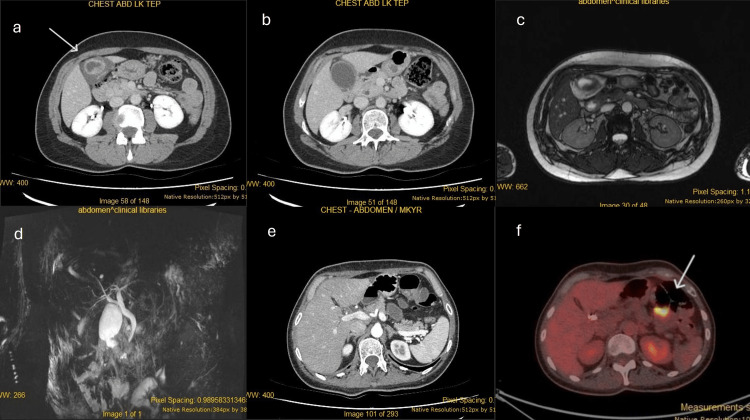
Imaging Pictures a, b: initial presentation CT scan; pictures c, d: MRI and MRCP scans; picture e: post-cholecystectomy dual-phase CT; picture f: PET CT scan post-cholecystectomy
